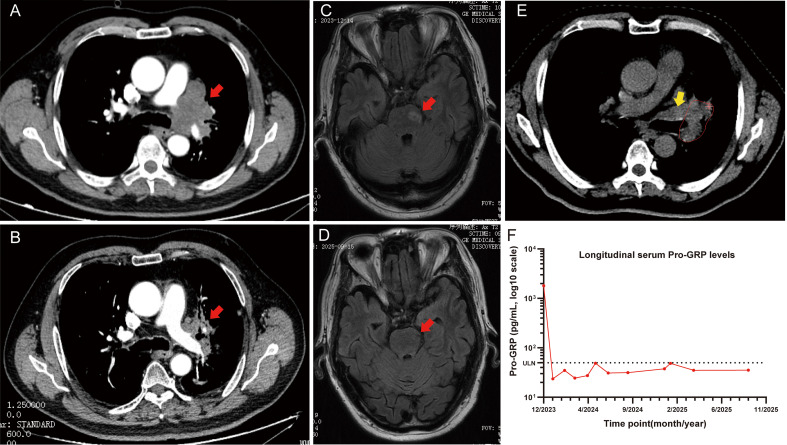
(A–D) Comparative imaging before and after treatment. (A) Baseline contrast-enhanced chest CT scan of the primary lung lesion. (C) Baseline contrast-enhanced brain MRI scan of the brainstem lesion. (B, D) show the most recent post-treatment follow-up images corresponding to (A, C), respectively. (E) Delineation of the gross tumor volume (GTV). The red contour outlines the primary GTV, While yellow arrows indicate the primary lung lesions that were not irradiated as a result of radiation field reduction (target volume shrinkage) within the lung. (F) Longitudinal changes in pro-gastrin-releasing peptide (pro-GRP) levels during the treatment course. X-axis: time point (month/year). Y-axis: Pro-GRP (pg/mL) displayed on a log10 scale. The dashed line indicates the upper limit of normal (ULN, 50 pg/mL). The graph was generated using GraphPad Prism software (version 10).
